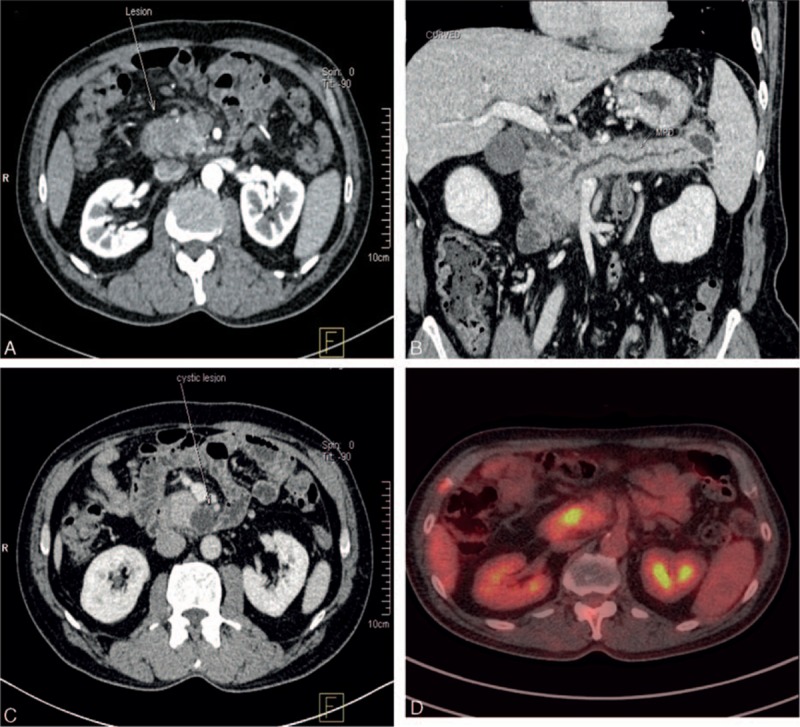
Imaging findings from the pancreatic tumor. (A) Computed tomography detected a 2 × 1.5 cm hypodense solid mass with an unclear margin in the head of the pancreas; (B) expanded primary pancreatic duct and the cystic low density in the tail of pancreas; (C) cystic low density in the uncinate process of the pancreas; (D) PET-CT revealed a malignant mass in the pancreatic head.
